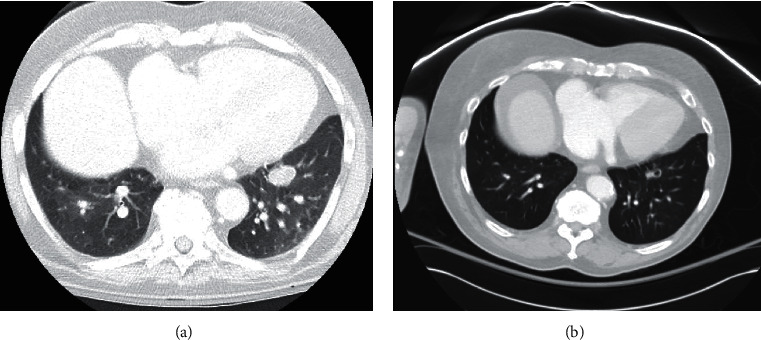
Chest CT. (a) pulmonary nodule on initial presentation; (b) pulmonary nodule on repeat imaging, 3 weeks after initial presentation.
